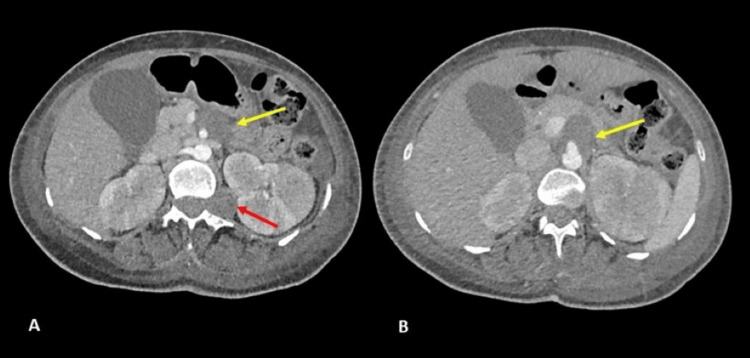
Contrast abdominal CT scan in arterial phase showing a mass (yellow arrow in A and B) with soft tissue density, without enhancement, and with well-defined borders, anterior to the celiac trunk. In addition, a lesion of similar characteristics of left paravertebral location, apparently in contact with the spinal canal and emerging from it (red arrow in A). Subsequently, the diagnosis of mesenteric schwannoma was made.
